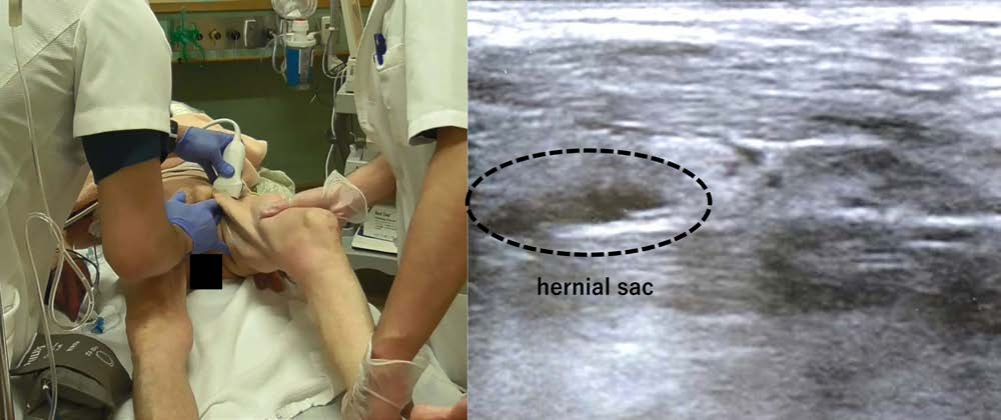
Step 3. The assistant places the hip and knee joints in an extended position. During this maneuver, the surgeon pushes the hernial sac more forcefully. The ultrasound image shows an empty hernial sac after this maneuver.
